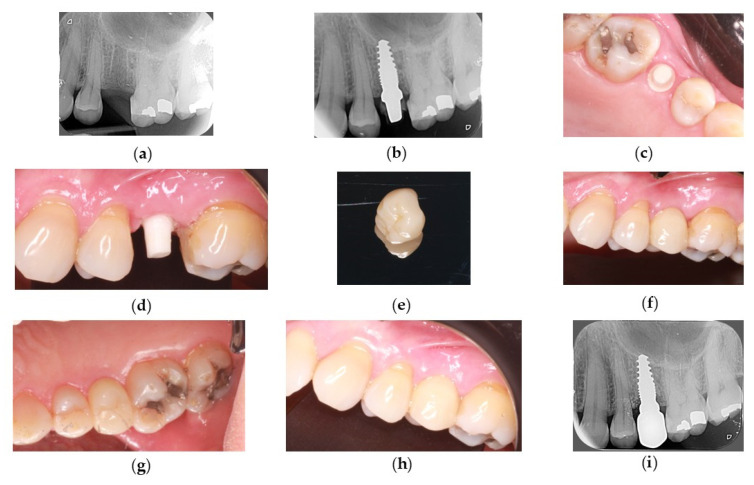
Complete clinical case with 3-year follow-up. (a,b) Periapical radiographs showing the post-extraction socket before implant placement and the tapered implant in place (Z-Systems Z5m(t) -4010). (c,d) Clinical images at the end of the osseointegration period. Note the health of the soft tissue. (e) Monolithic zirconium permanent crown manufactured using CAD/CAM technology. (f,g) Intraoral images of the crown in place. (h) Clinical image taken 3 years after placement. Note the excellent health of the soft tissue and integration of the restoration. (i) Periapical X-ray taken 3 years post-loading showing stable bone tissue.
